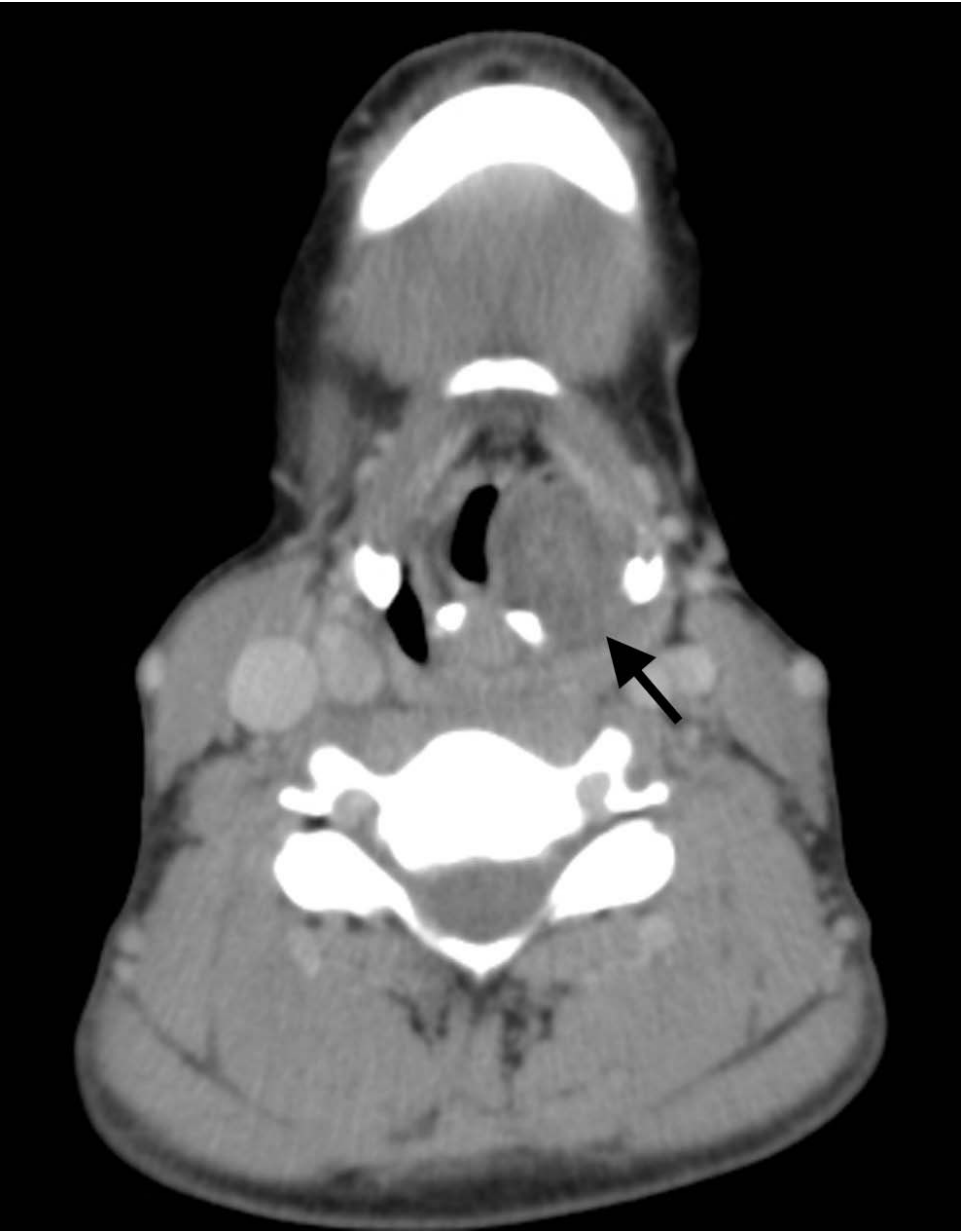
CT image of the well defined, hypodense, round to oval submucosal mass in the left supraglottic larynx (arrow).
